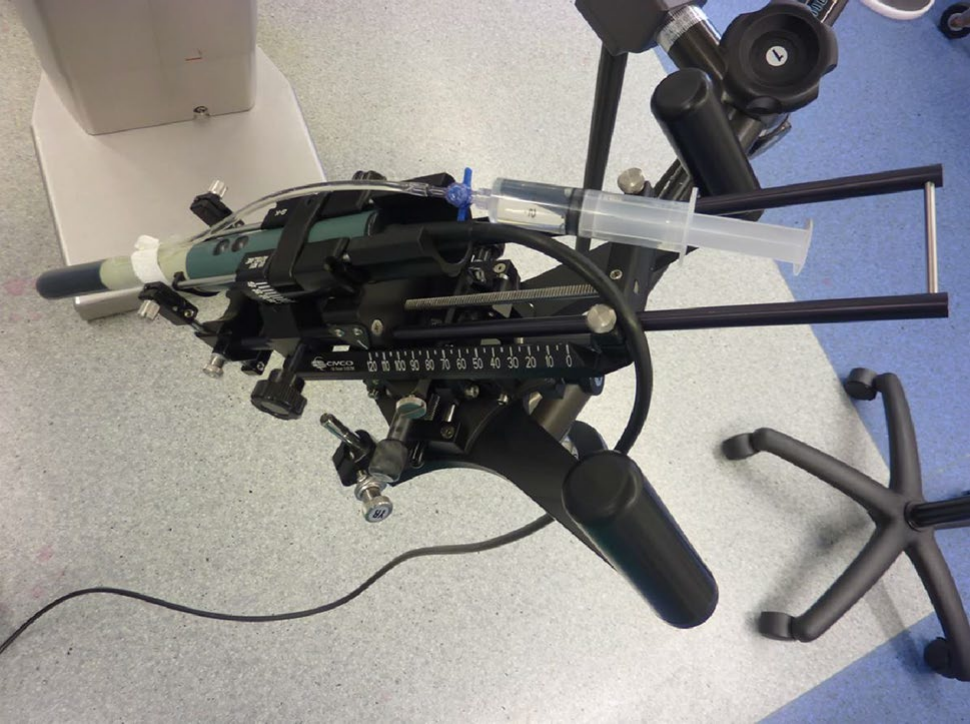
The ultrasound probe, the balloon and the brachystepper device
